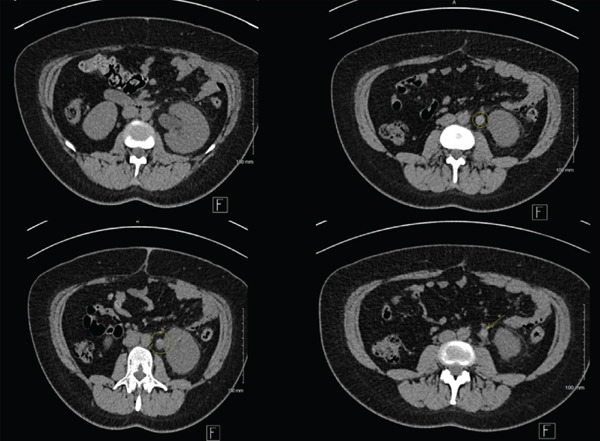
Noncontrast CT abdomen and pelvis showing left hydroureter and hydronephrosis in addition to an ipsilateral lesion of the proximal ureter.
